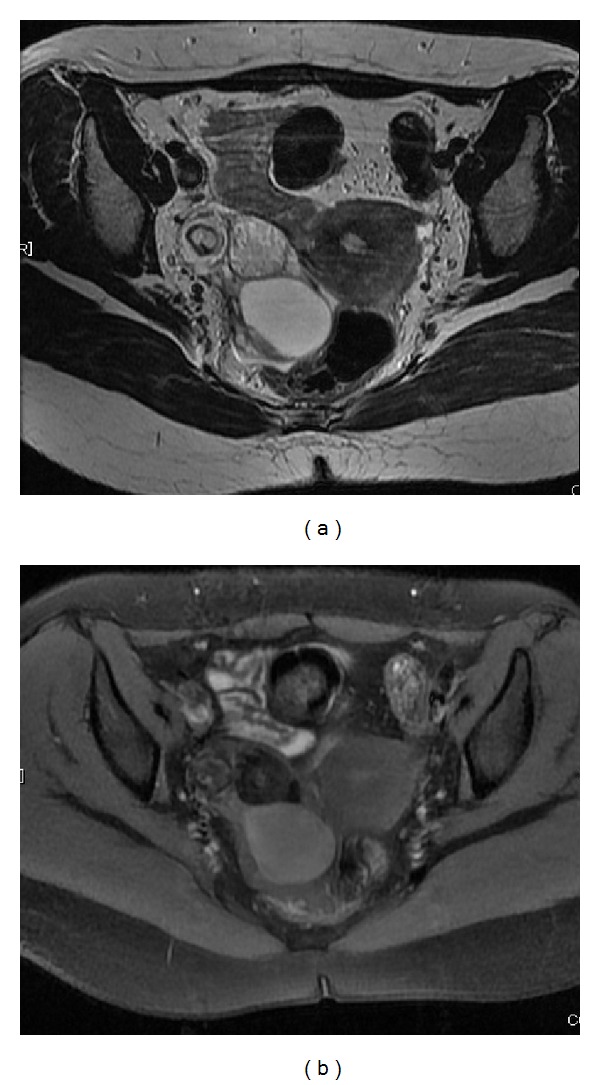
Right ovarian cyst with fat in the cystic cavity before the third surgery. The cyst size was the same as the cyst size before the second surgery. (a) Axial T2-weighted image shows high-signal intensity cyst. (b) Fat-saturated T1-weighted image shows a signal drop on the cyst.
